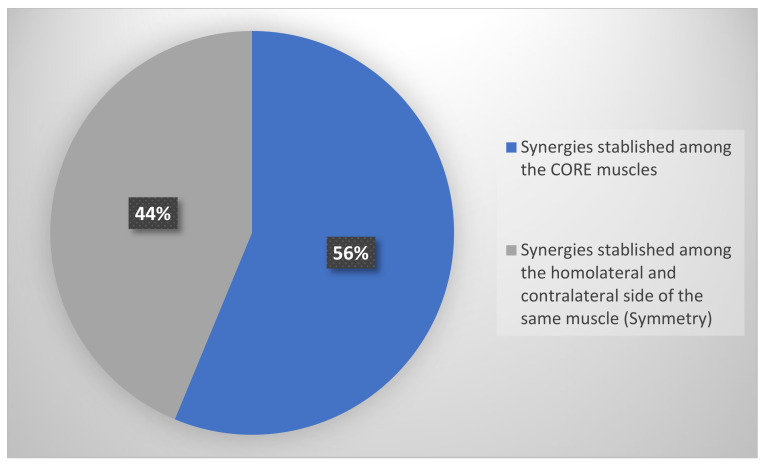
Types of synergies established with ultrasound.
